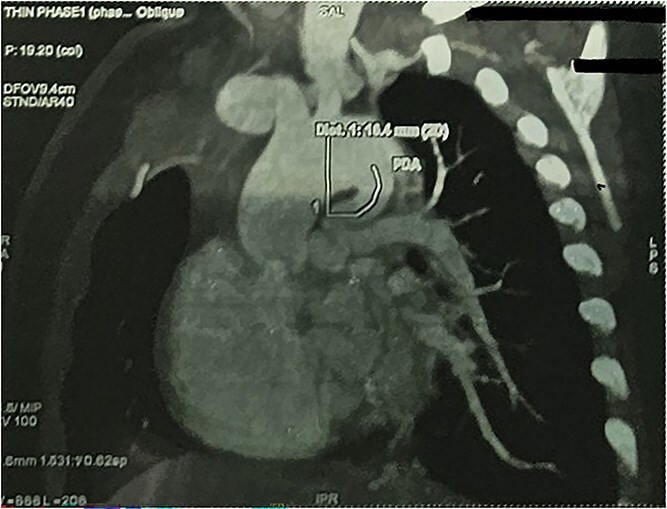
CTA demonstrating long tortuous PDA supplying the pulmonary circulation.
